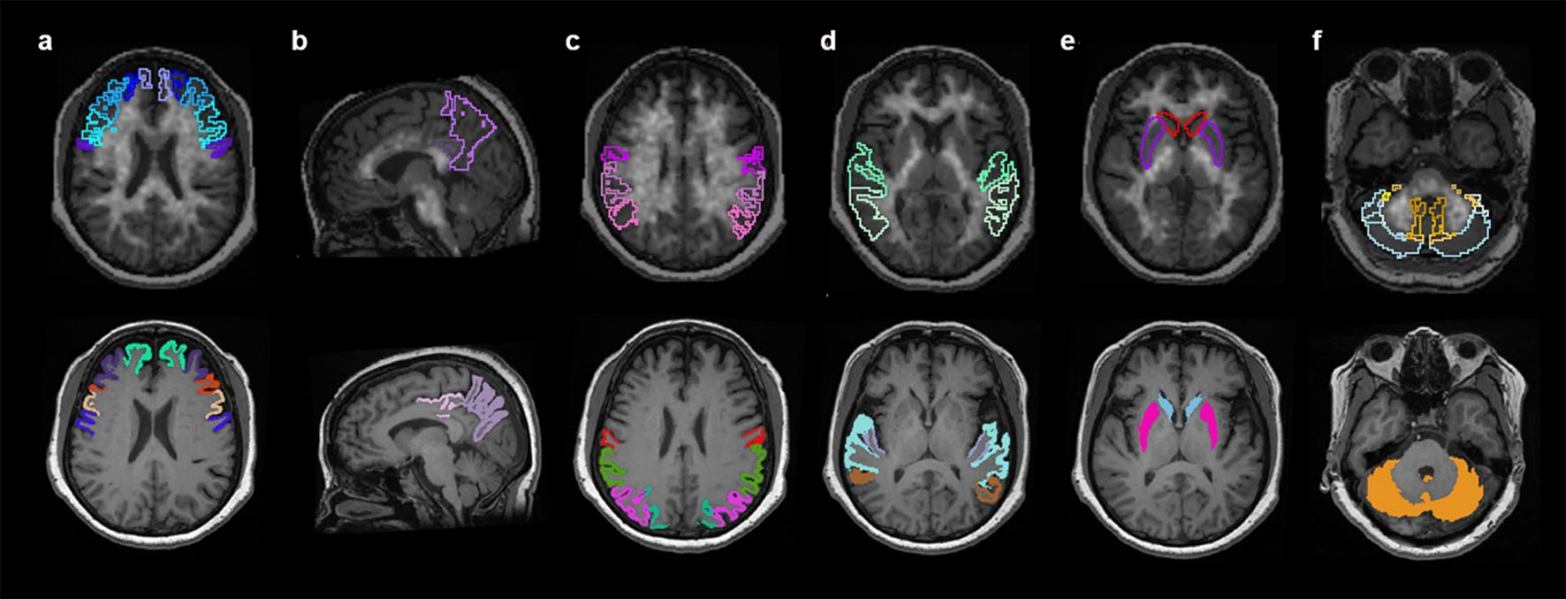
Representative images for contours of volumes of interest (VOIs). The VOIs for the frontal lobe (a), precuneus/posterior cingulate (PC/PCC) (b), parietal lobe (c), temporal lobe (d), striatum (e), and cerebellar grey matter (f) with coloured contours are automatically set in co-registered PET-MR images using the AAL-VOIs atlas provided by PMOD (top row) and MR images fed to Heuron (bottom row).
